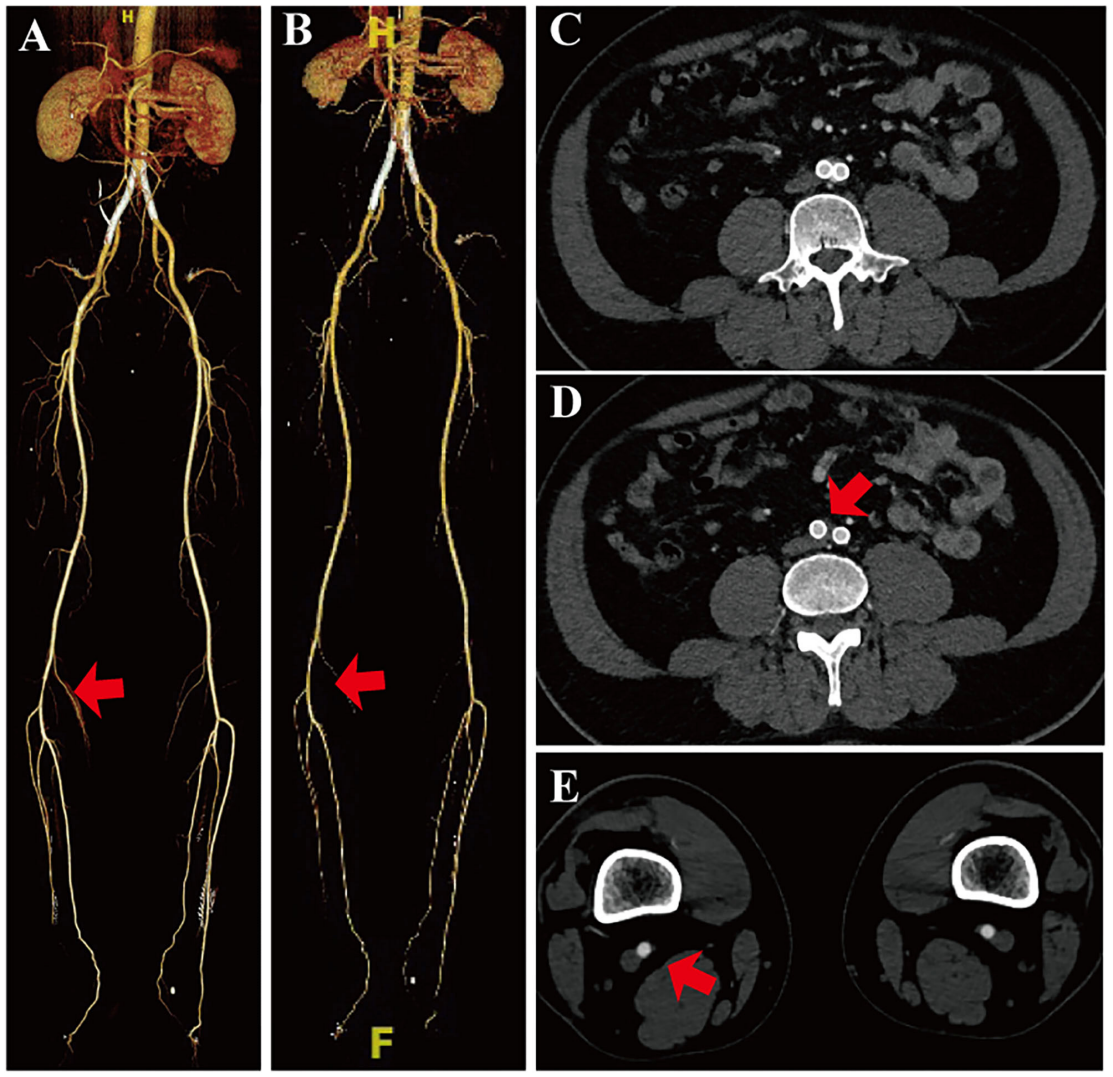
Follow-up CTA of the same patient. (A) CTA shows the patency of the bilateral common iliac artery and the right popliteal artery after 6 months (red arrow). (B) CTA shows the patency of the bilateral common iliac arteries and the right popliteal artery after 18 months (red arrow). (C–E) Cross-section of CTA of 18 months.
